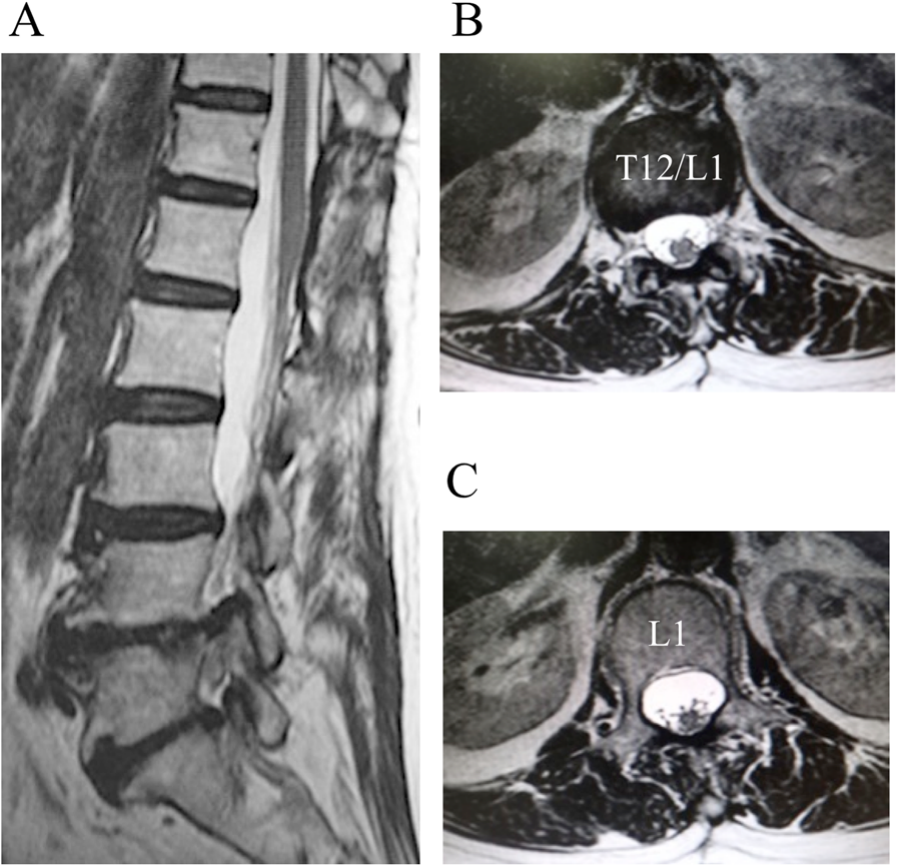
Magnetic resonance imaging (MRI) of the thoracolumbar spine 2 years after surgery. MRI of the thoracolumbar spine obtained 2 years after surgery showing complete resection of the tumor, and there was no recurrence of the tumor. Views: a T2-weighted sagittal, b T2-weighted axial at the T12–Ll, and c T2-weighted axial at L1
